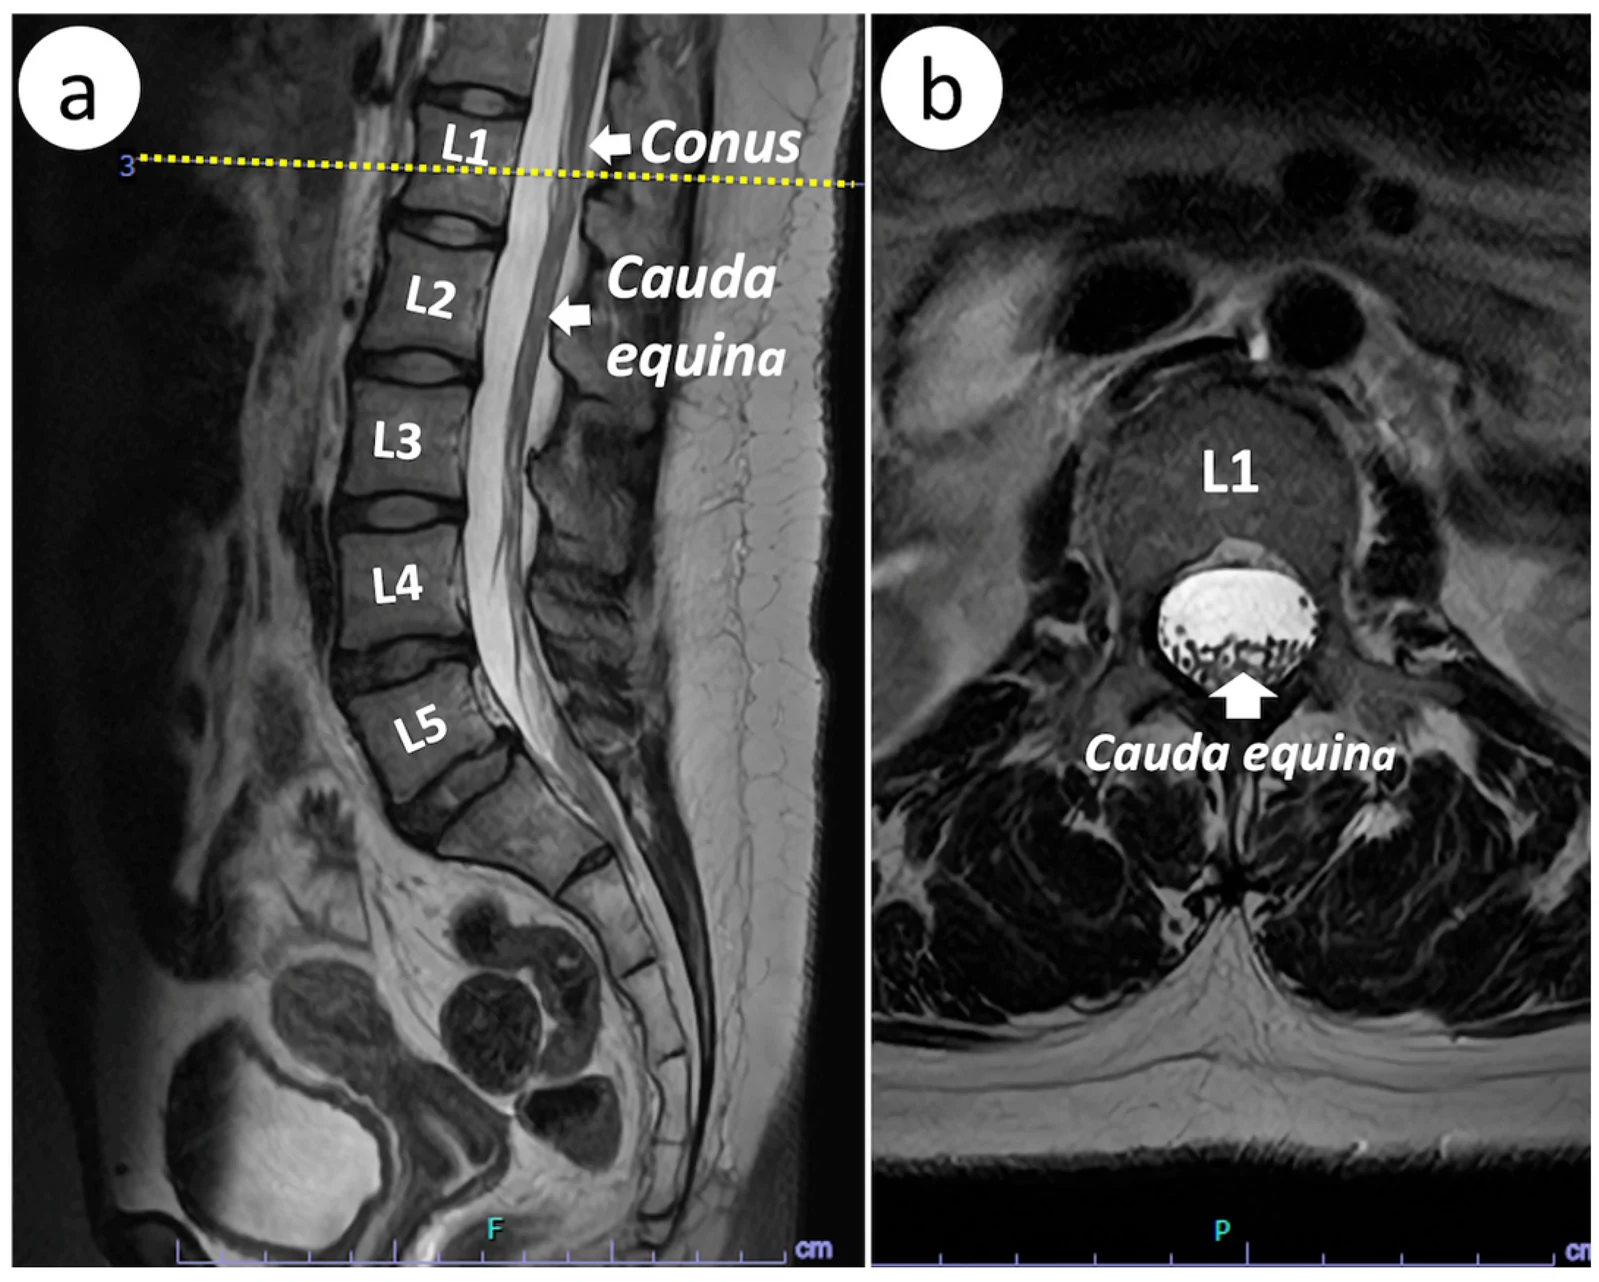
Lumbar magnetic resonance imaging. (a) Sagittal T2-weighted magnetic resonance imaging demonstrating a normally positioned conus medullaris (Conus) terminating at the L1 level without evidence of tethered cord. (b) Axial T2-weighted magnetic resonance imaging obtained at the L1 level (yellow dotted line in panel (a)).
